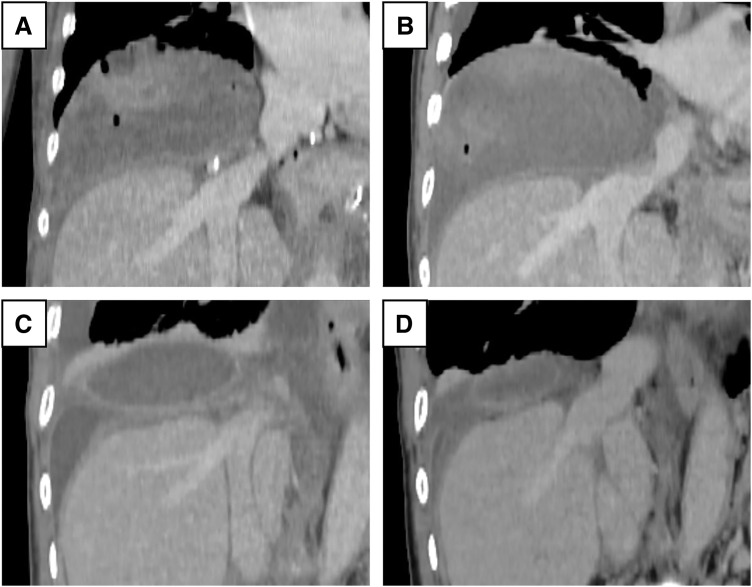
Serial coronal CT images demonstrating the natural course of the pneumatocele. (A) CT image obtained immediately postoperatively. (B) CT image at 2 weeks postoperatively. (C) CT image at 3 months postoperatively. (D) CT image at 6 months postoperatively. The pneumatocele gradually decreased in size over time, indicating a tendency toward spontaneous resolution without intervention.
